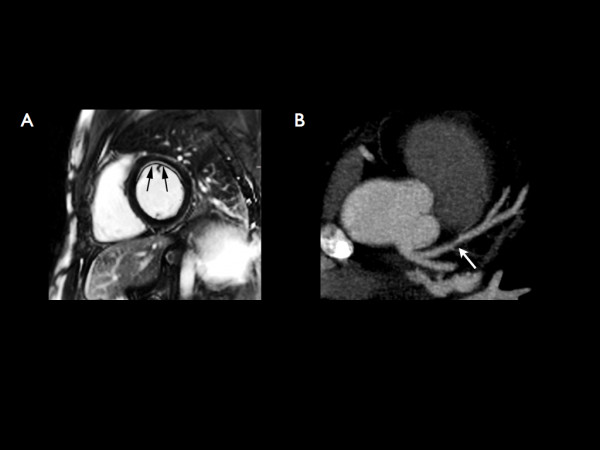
A) Short axis phase sensitive IR-prepared T1-weighted TurboFLASH MR image demonstrating subendocardial enhancement involving the anterior wall of the LV at baseline, prior to the marathon (arrows).B) Curved planar reformation of a contrast-enhanced CT angiogram in the same patient demonstrating a >70% stenosis of the LAD artery (arrow).
